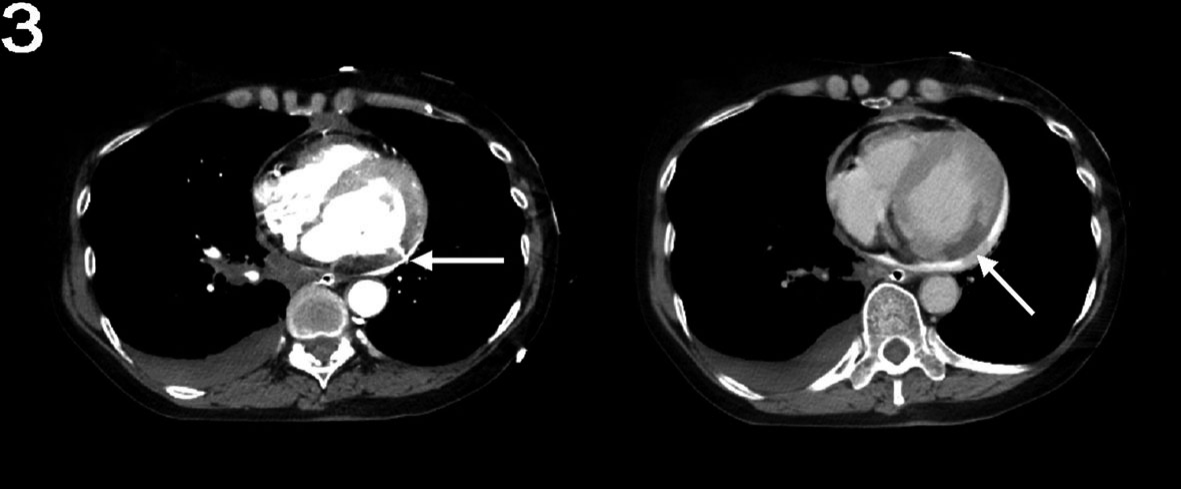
Left: Early images with a CT scan displaying a small rupture (arrow) in the posterolateral wall of the left ventricle with the presence of a hemopericardium, confirming the diagnosis of free wall rupture. Right: Delayed CT images (60 seconds after initial images) showing a small, hypodense area of infarction (arrow) in the inferolateral wall, with greater degree of contrast extravasation relative to the early images, suggesting active bleeding into the pericardial sac.
